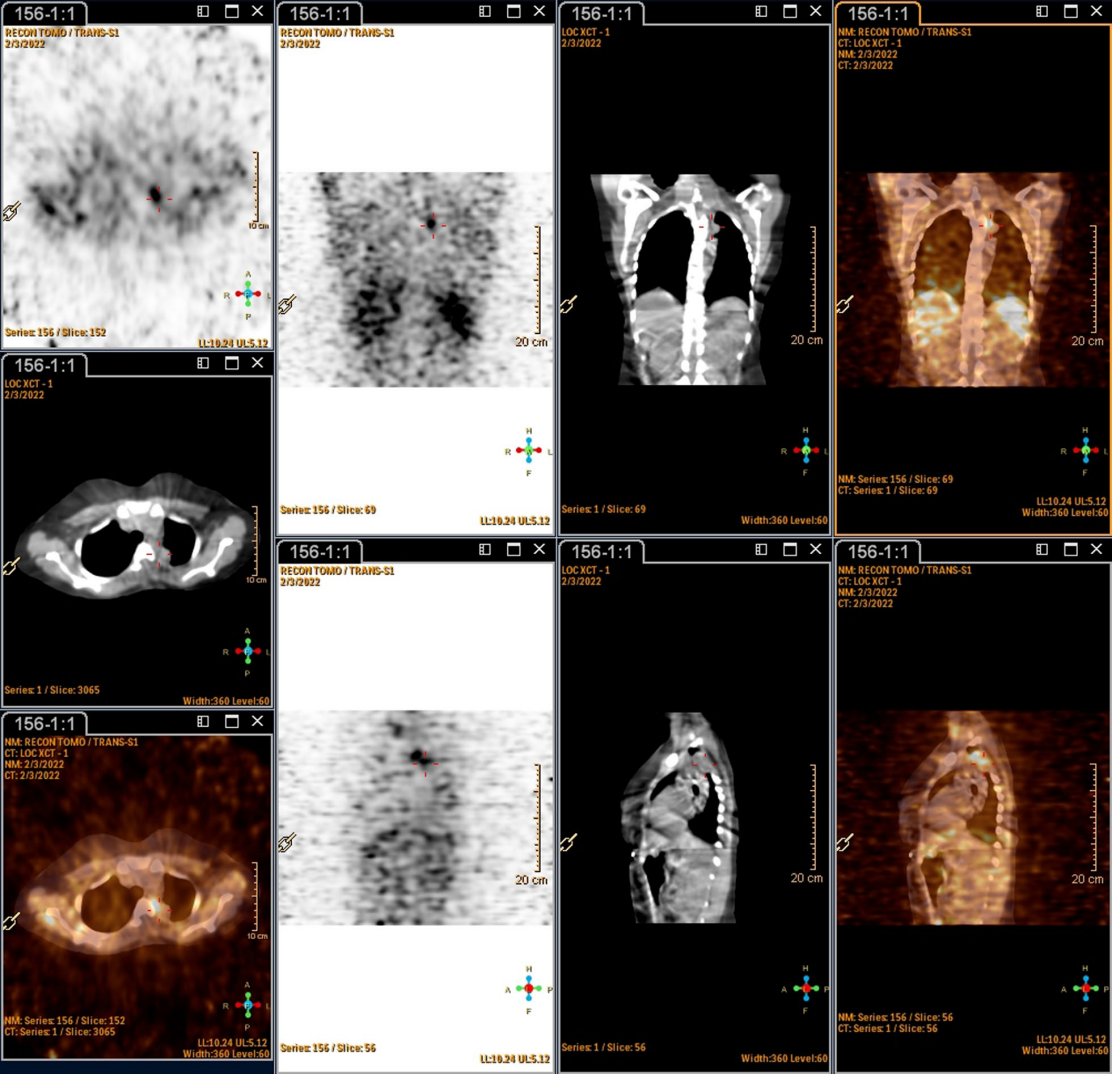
Whole body planar anterior and posterior MIBG images showing focal uptake in the left upper thorax, in the paraspinal region, corresponding to the mass seen on CT
